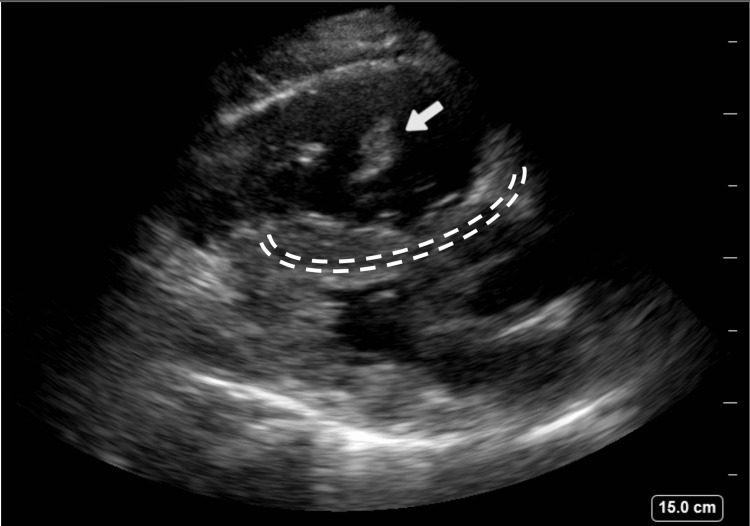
Sonosite XPorte with phased array probe in a parasternal long axis view demonstrating a mobile hyperechoic structure (white arrow) in the right ventricle. Interventricular septal bowing from right to left is visible (white dashed lines).
